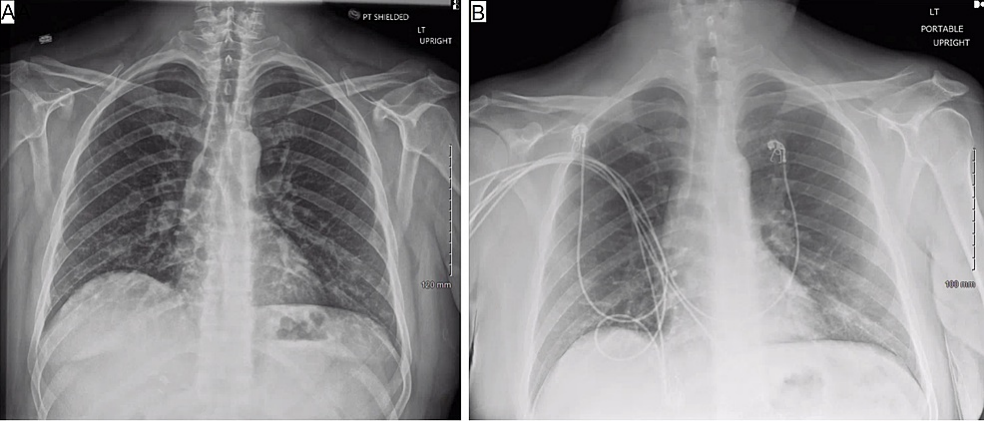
Chest X-ray of the patients at the initial visit (Figure 1A) and second visit (Figure 1B)
